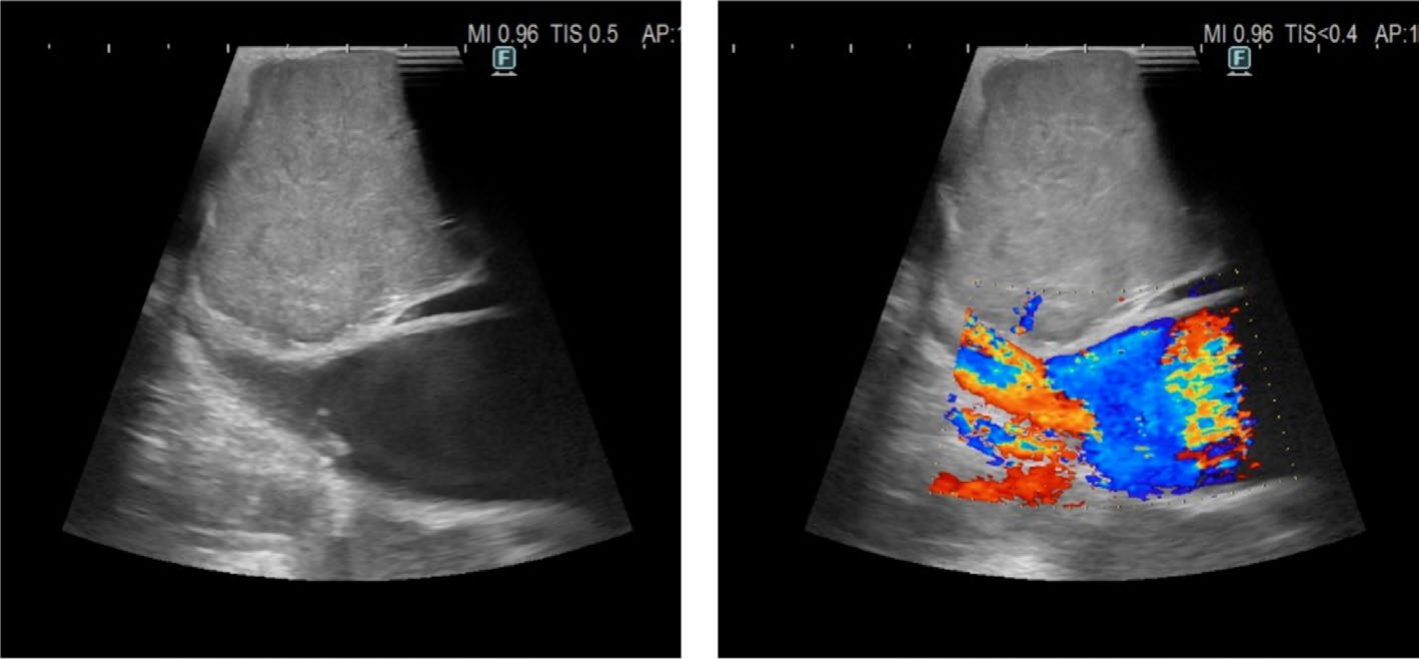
The ultrasound characteristics of mediastinal masses were: Large hypoechoic lesions, inhomogeneous echostructure, increased vascularization in the intra‐ and perinodular color Doppler, presence of hyperechoic spots, and contextual anechoic liquefactive areas.
